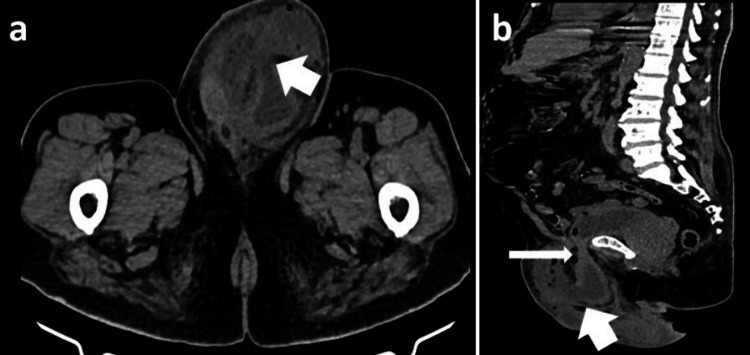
NCCT of abdomen and pelvis: (a) axial section showing a discontinuity in the wall of the herniated urinary bladder (short white arrow); (b) sagittal section showing herniation of the urinary bladder into the left inguinal canal (long white arrow) with a discontinuity in the wall of the herniated urinary bladder (short white arrow) NCCT, non-contrast CT
